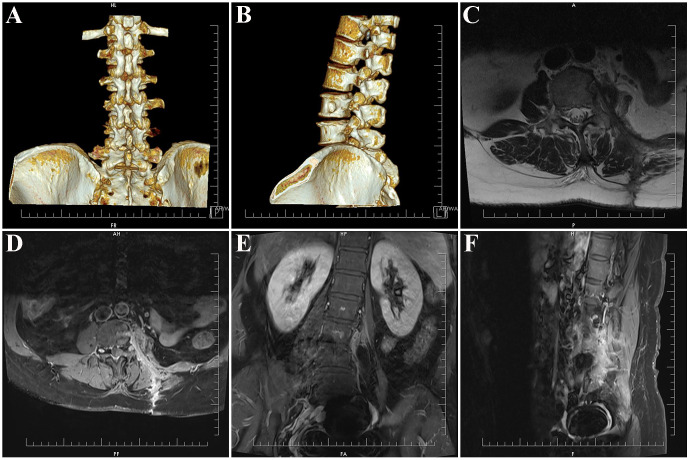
Postoperative three-dimensional CT and three-month follow-up MRI. (A, B) Three-dimensional CT reconstruction showing partial resection of the left L3 and L4 transverse processes, with preservation of other bony structures. (C) Postoperative T2-weighted image demonstrating the surgical corridor through the Weaver intermuscular plane. (D–F) Postoperative contrast-enhanced T1-weighted axial, coronal, and sagittal images confirming complete tumor resection.
